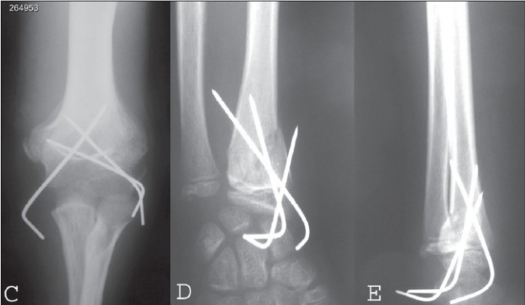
(C) AP X-ray of elbow shows precutaneous pinning of supracondylar fracture with callus formation. (D) AP and lateral X-ray (E) of distal forearm and wrist shows percutanous pinning of distal radius fracture
